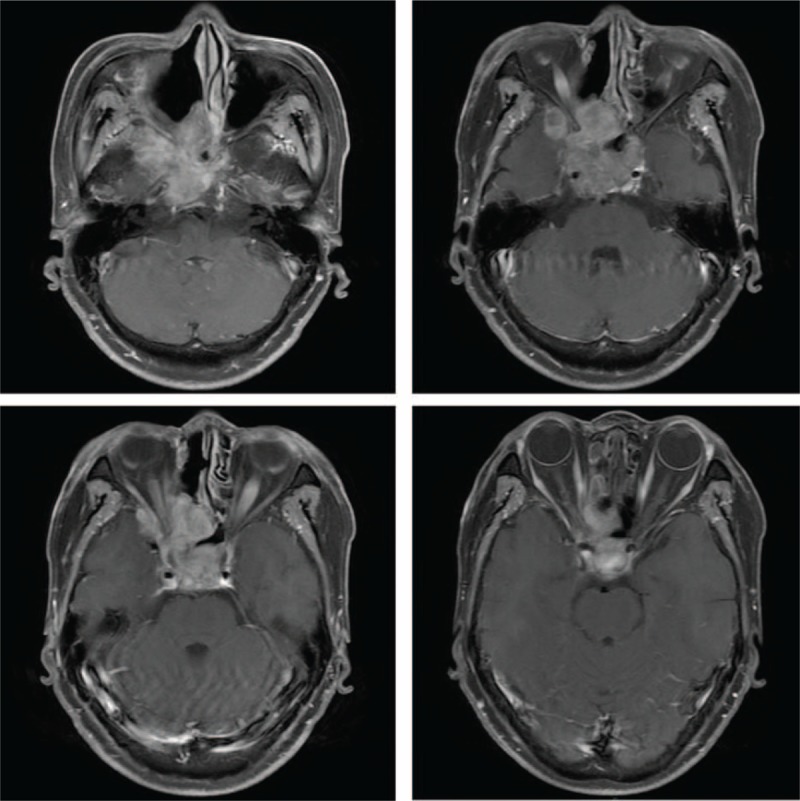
T1-weighted image with contrast enhancement showing a soft tissue mass measuring 5.0 × 4.3 × 6.1 cm in the saddle area and 2 sided sphenoid sinus.
